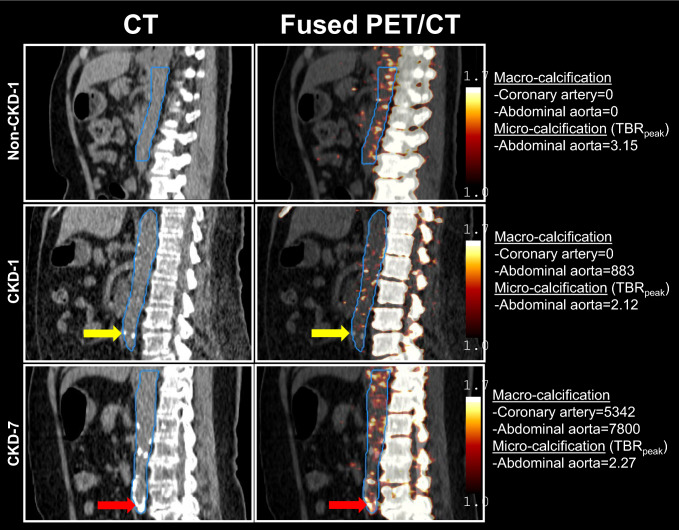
Representative sagittal images of macro- and micro-calcification in abdominal aorta from 1 participant without CKD and 2 participants with CKD. VOIs for abdominal aorta are outlined in blue. Low dose CT imaging shows macro-calcification indicated by radiopacity. Fused PET/CT imaging shows micro-calcification indicated by high ^18^F-NaF uptake and represented by peak tissue-to-background ratio (TBR_peak_). Yellow arrows indicate lesions with macro-calcification on CT without any ^18^F-NaF uptake on PET. Red arrows indicate colocalized lesions of macro-calcification and ^18^F-NaF uptake. Color scale indicates the degree of radioactivity in TBR_peak_
